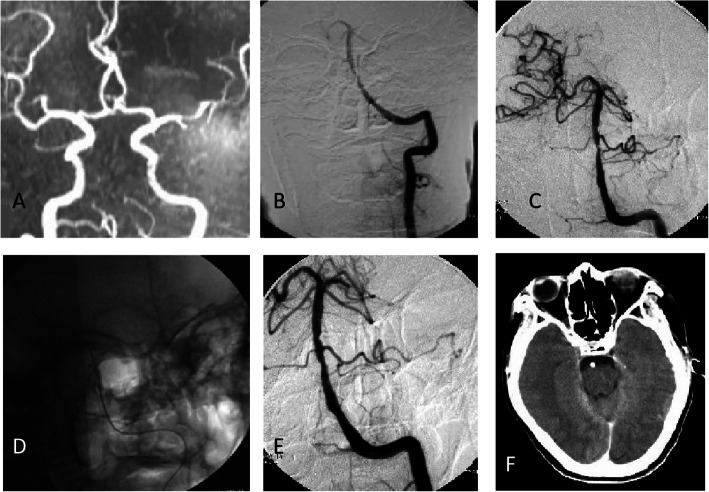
Management of subacute thrombosis in the stent. a Preoperative MRA images showed subacute occlusion after basilar stenting. b Preoperative DSA images showed acute occlusion after basilar stenting. c-d Intraoperative, intra-arterial thrombolysis and balloon dilation. e Postoperative, subacute occlusion of basilar artery was recanalized. f Postoperative CT images showed no intracranial hemorrhage
